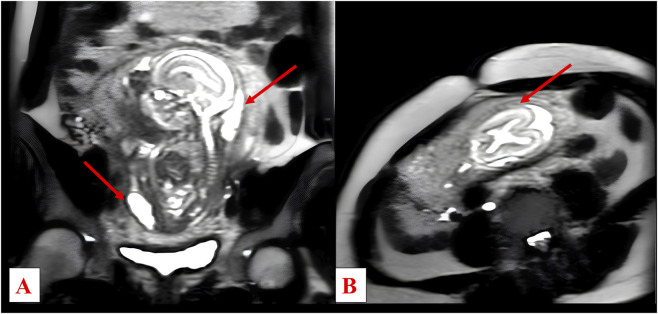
Low-resolution T2-weighted MRI scans. (A) Sagittal plane of the fetus showing oligohydramnios/anhydramnios (arrows). (B) Coronal plane of the fetal head showing a slightly reduced biparietal diameter and a morphological tendency toward scaphocephaly, with the cranial contour highlighted by an arrow.
